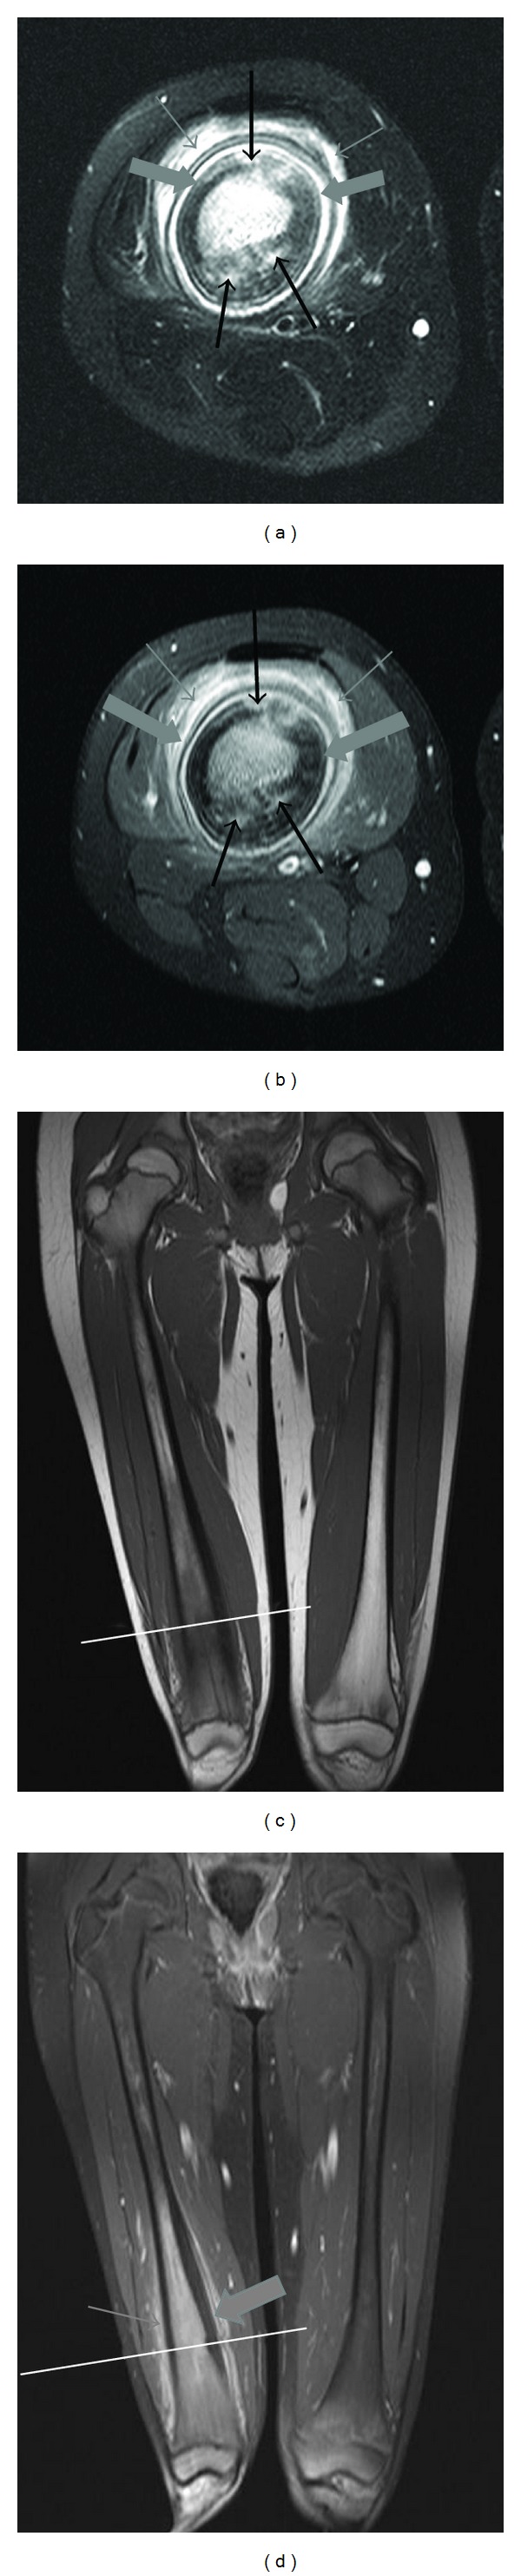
Axial (a-b) MRI through the right thigh with coronal (c-d) MRI images through both thighs. Displayed axial images are from the level of the white lines in (c) and (d). Sequences are as follows: (a) axial T2 fat saturated (FS) (TE 93 ms. TR 5670 ms.), (b) axial T1FS with gadolinium (TE 17 ms. TE 730 ms.), (c) coronal T1 (TE 14 ms. TR 543 ms.), and (d) coronal T1FS with gadolinium (TE 14 ms. TR 460 ms.). The axial T2 FS image displays the edematous high signal in the medullary space, patchy high signal cortical lesions (black arrows), cortical thickening and periosteal reaction (thick grey arrows), and subtle surrounding soft tissue edema (thin grey arrows). Coronal T1 (c) shows the low signal within the medullary space, a characteristic feature of involvement. After gadolinium (b,d) there is intense enhancement of the marrow, periosteum (thick grey arrows), with some enhancement also seen in the bordering musculature (thin grey arrows).
